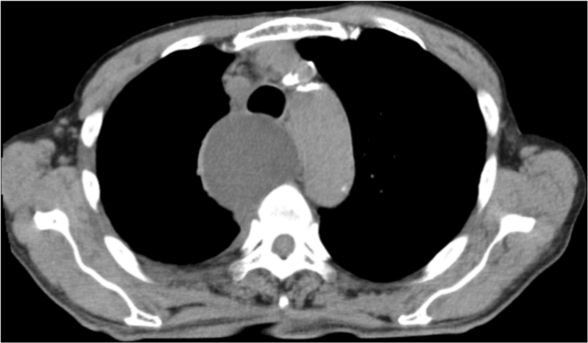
Chest computed tomography showing a large mediastinal fluid collection surrounded by a thick capsule that is compressing the trachea and esophagus
